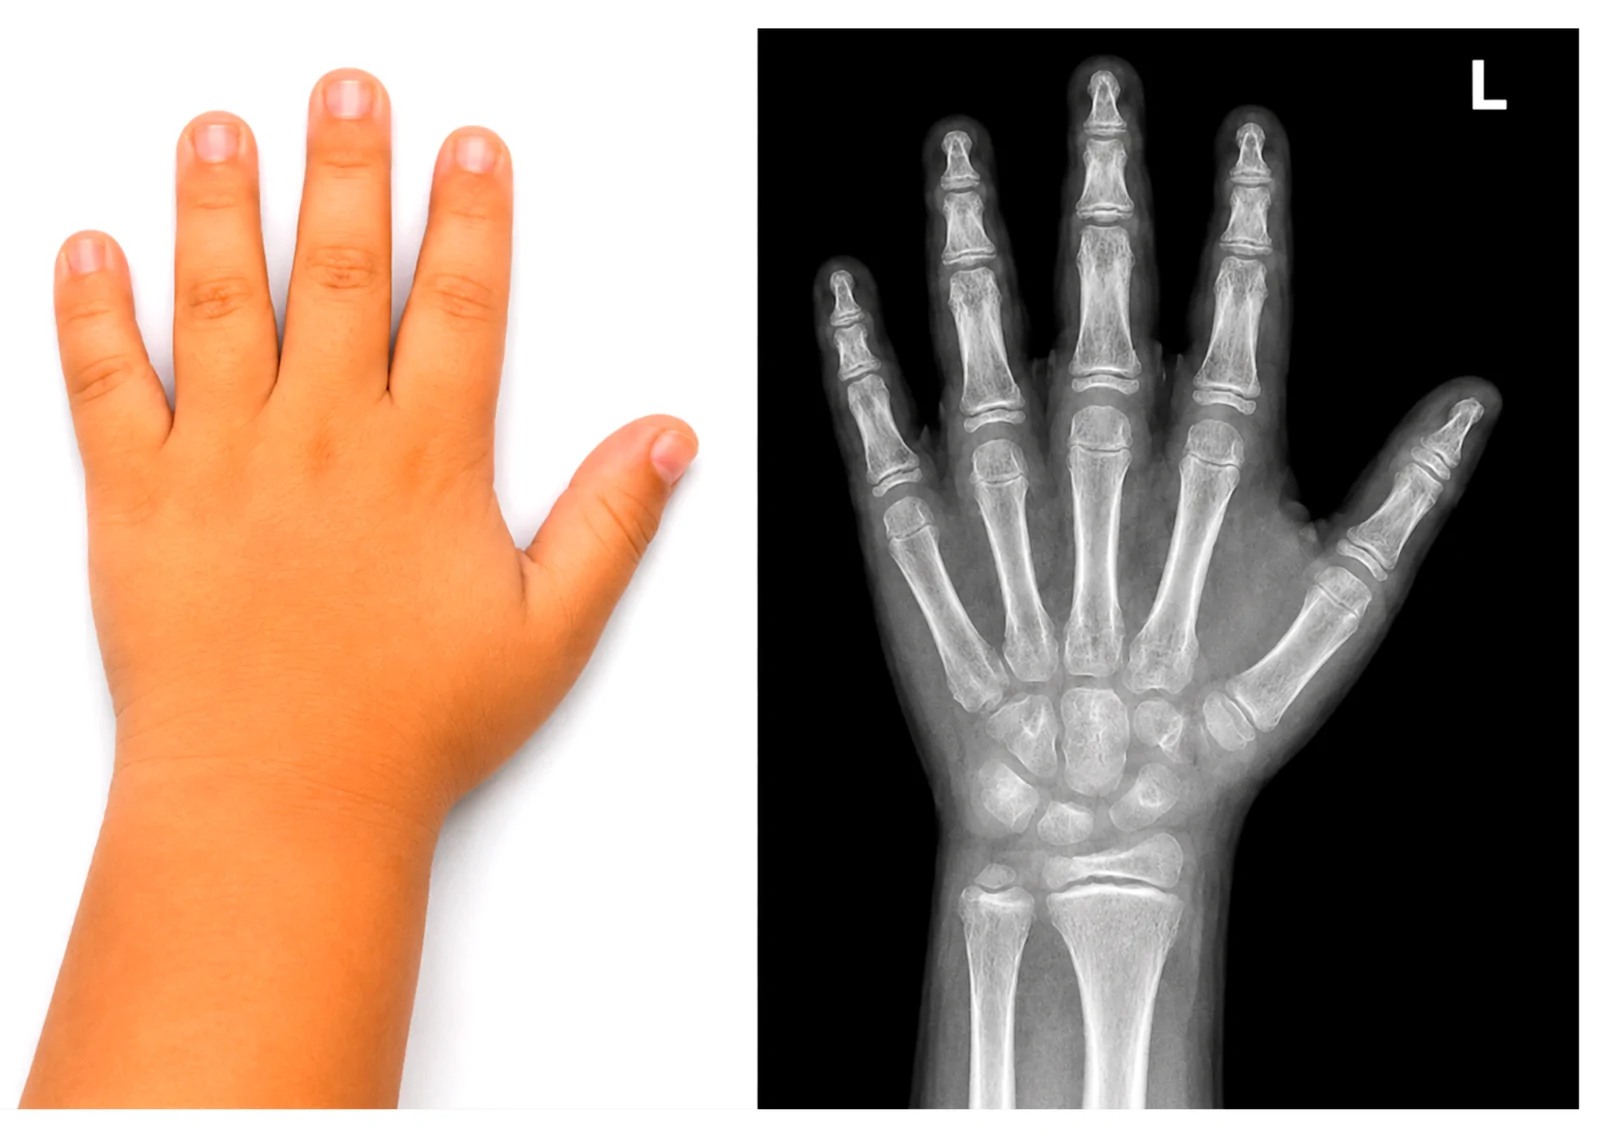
Clinical and radiographic appearance of the left hand. Dorsal image of the left hand (left) and its corresponding PA-HW X-ray (right). The images show shortening of the middle phalanges and brachydactyly, findings consistent with BDA1 linked with a pathogenic *IHH* variant. Radiographic evaluation using GPA showed delayed skeletal maturation relative to CA. Source: own work.
